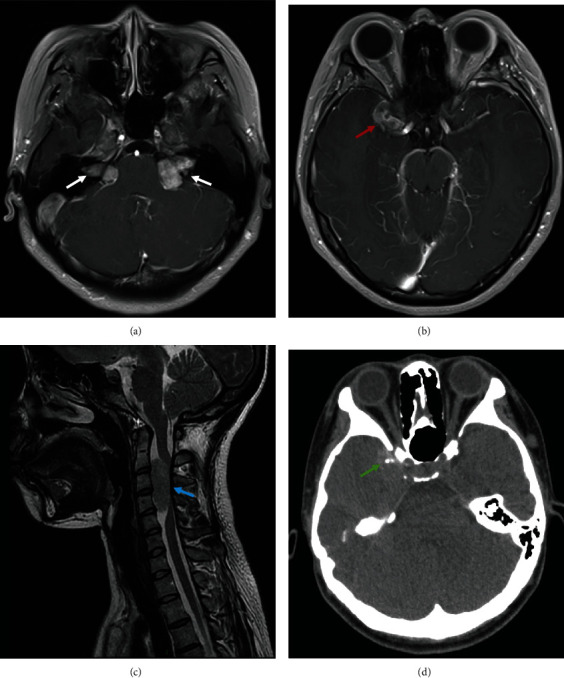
Neuroimaging of the patient with neurofibromatosis type 2. (a) Bilateral solid masses in the cerebellopontine angles on axial enhanced MRI (white arrows). (b) Axial enhanced MRI shows a parasellar lesion (red arrow). (c) Sagittal T2-weighted MRI of the spine shows a focal hypointense abnormality at the C2-C4 level (blue arrow). (d) Brain CT shows calcified lesions in the right temporal lobe (green arrow) and normal optic nerves.
